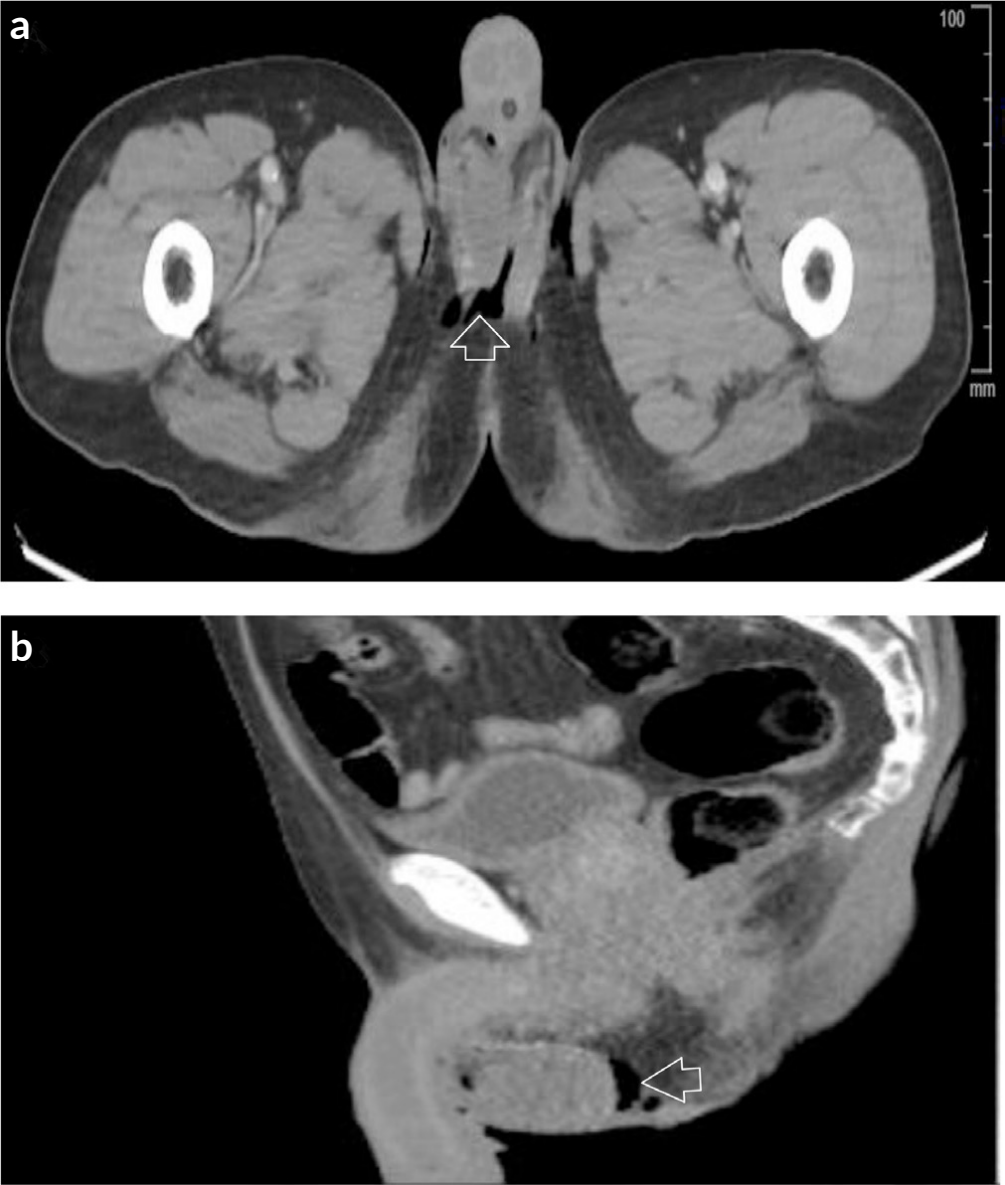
Contrast-enhanced CT scan of the abdomen and pelvis. (a): Axial section. (b): Sagittal view. Post surgical changes at the level of scrotal bags with scrotal emphysema (arrow), no adenopathies or distant metastases are observed.
